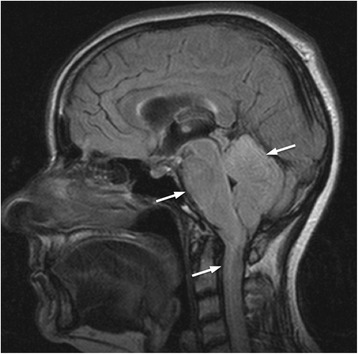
Diffuse T2/FLAIR hyperintensity in cerebellum, brainstem and cervical spinal cord (*arrows*)
